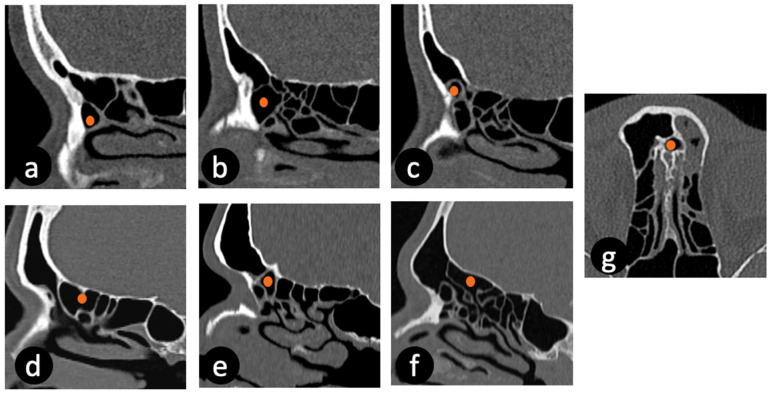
Frontal recess cell types according to the International Frontal Sinus Anatomy Classification on sagittal (a–f) and axial (g) computed tomography images: (a) aggar nasi cell, (b) supra aggar cell, (c) supra aggar frontal cell, (d) supra bulla cell, (e) supra bulla frontal cell, (f) supra orbital ethmoid cell, and (g) frontal septal cell type. In each image, the orange dot denotes the position of the specified cell type.
